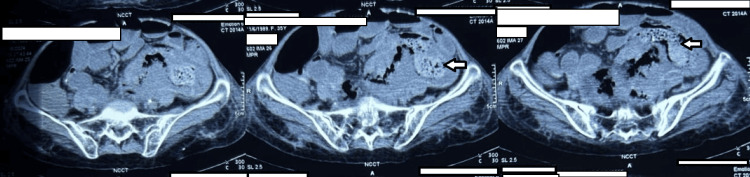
A non-contrast CT of the abdomen showing sigmoid colo-colic intussusception with an ill-defined lesion noted in the intussuscipiens. Panel 1: Cross-section showing sigmoid colo-colic intussusception. Panel 2: The arrow points at the ill-defined lesion seen in the intussuscipiens. Panel 3: The arrow points at the ill-defined lesion seen in the intussuscipiens.
